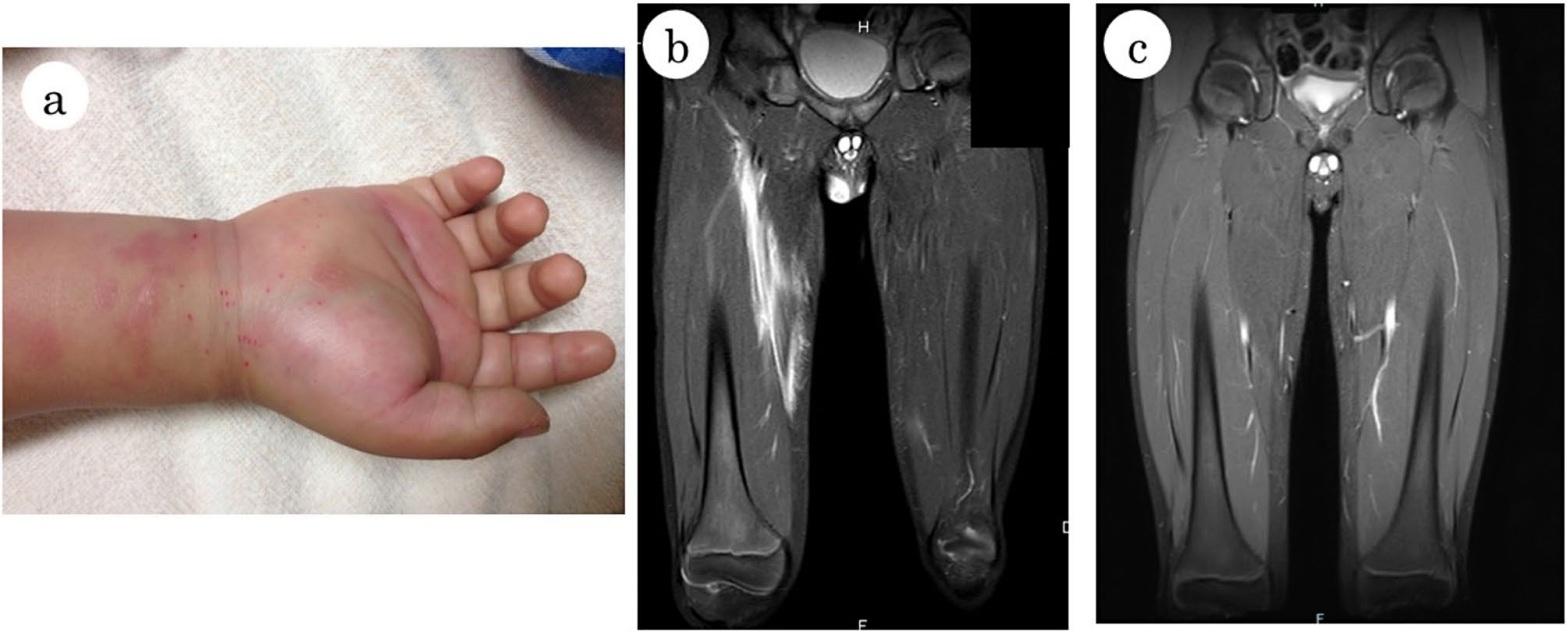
Macroscopic skin manifestations at the initial onset and MRI findings before and after the treatment during recurrence of the present patient. a: Skin swelling with erythema at the initial onset on the right upper limb following an abrasion injury on the right wrist. b: MRI of the lower extremities showing a high signal area in the right thigh on short tau inversion recovery. c: After the treatment, the high signal area in short tau inversion recovery disappeared
